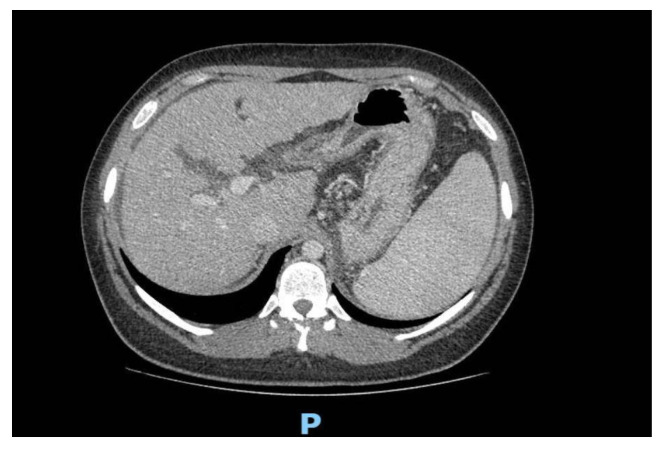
Abdominal computed tomography of Patient n°1. Many cirrhotic nodules are evident as hyper-density areas in the enlarged liver. LEGEND: P = posterior.
